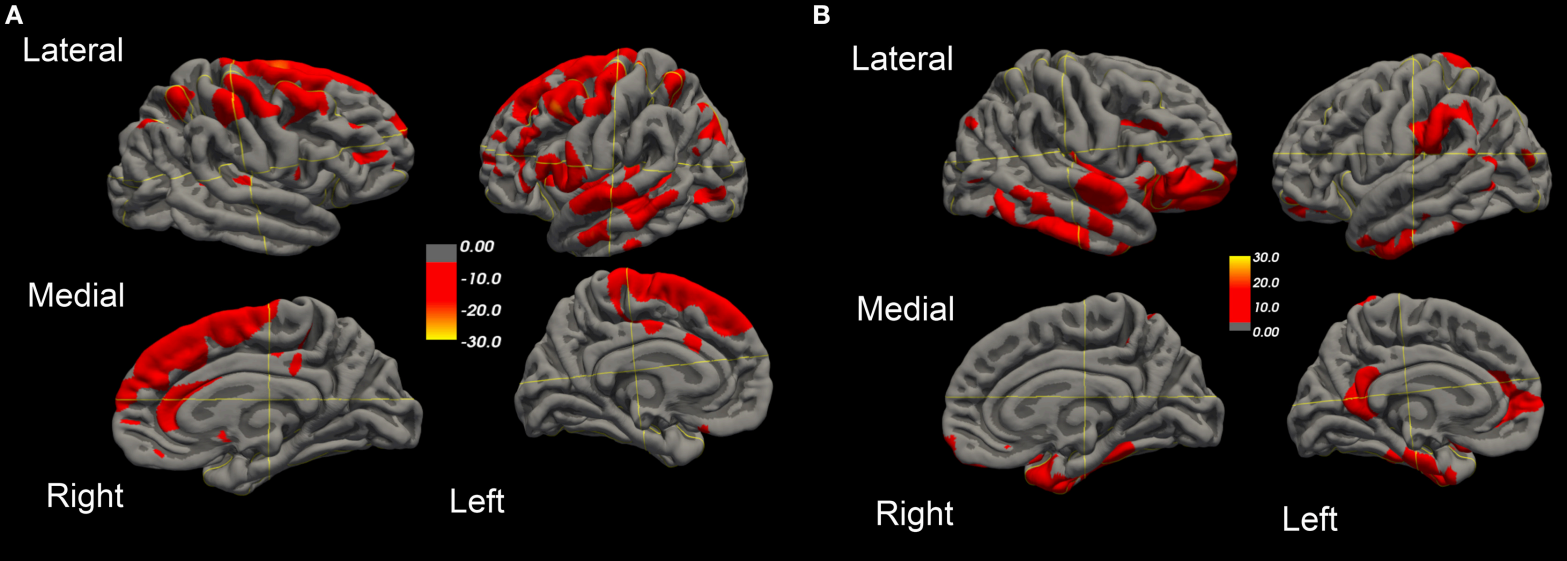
Surface-rendered MRI of right and left hemispheres SPC values of cortical surface area decrease (A) or increase (B) in the ALS-FTD patient over 20.4 months.
